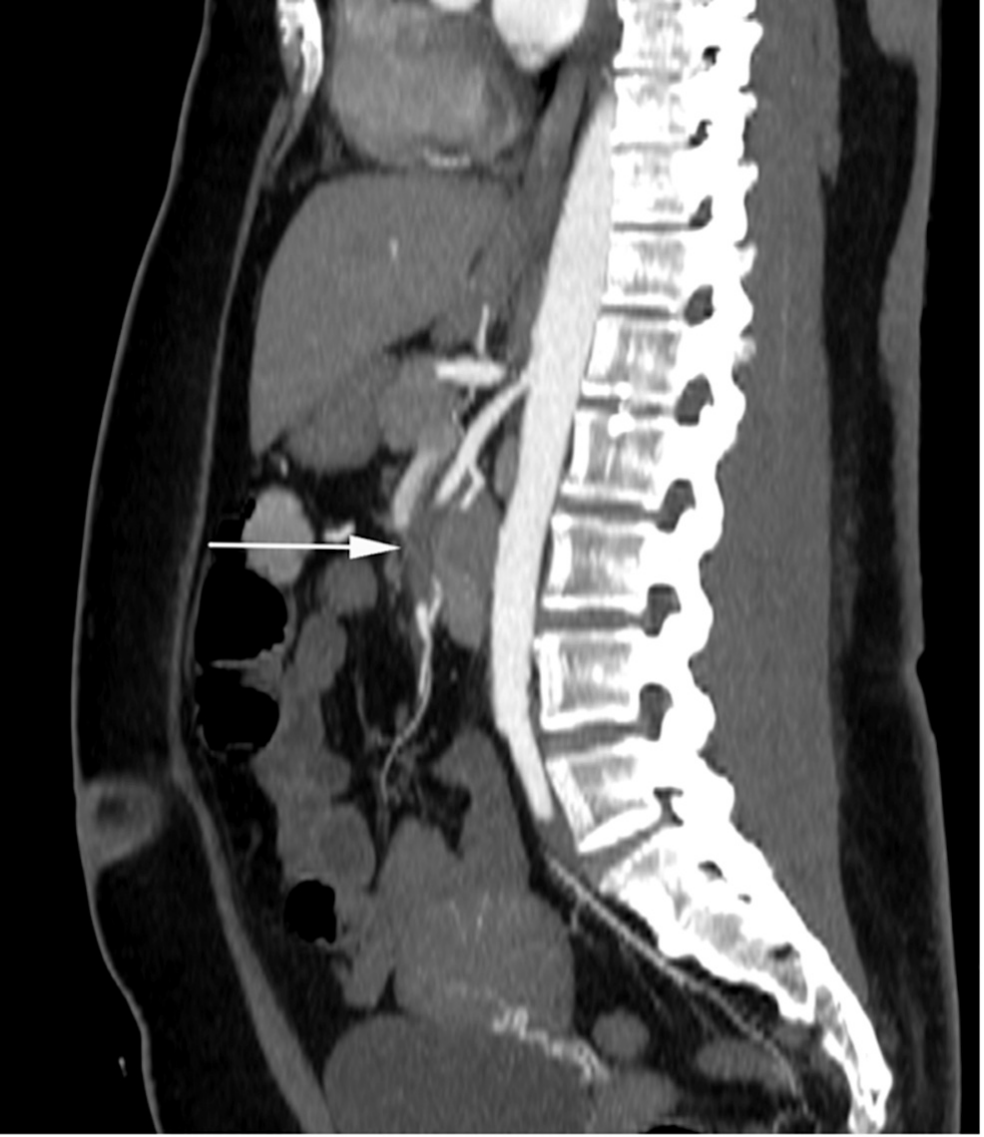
Sagittal reformatted, maximum intensity projection (MIP), post intravenous contrast computed tomography White arrow demonstrates occlusive thrombus in the mid/distal portion of the superior mesenteric artery (SMA).
